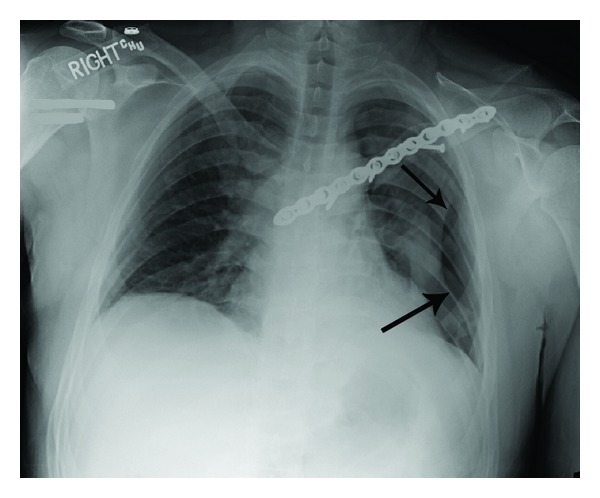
Chest radiograph taken in the recovery room after surgery. The arrows indicate the pneumothorax.
